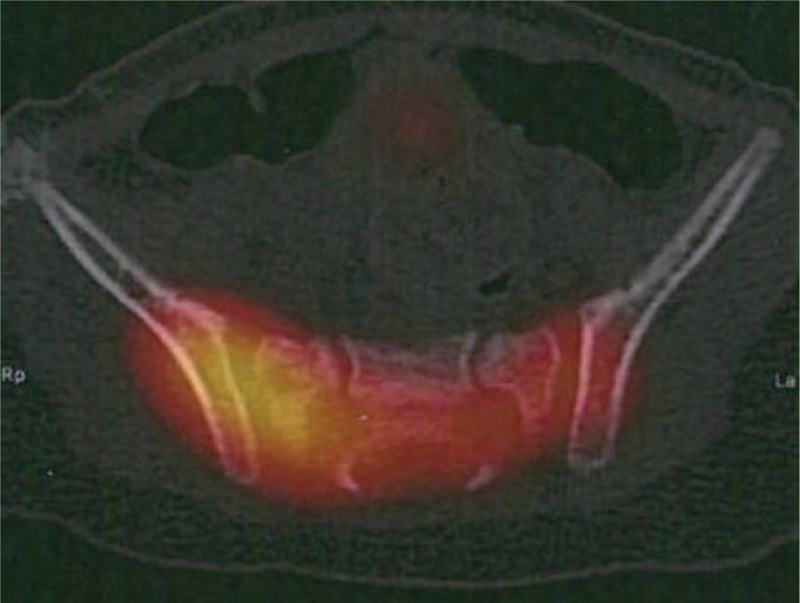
Axial view of SPECT. Hypermetabolism of the right sacroiliac joint.
